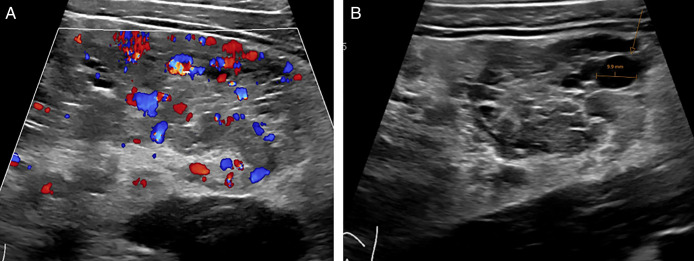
Ultrasound images with color Doppler (A) and without Doppler (B) of the marked thickened sigmoid colon, showing massively dilated veins in the bowel wall.
